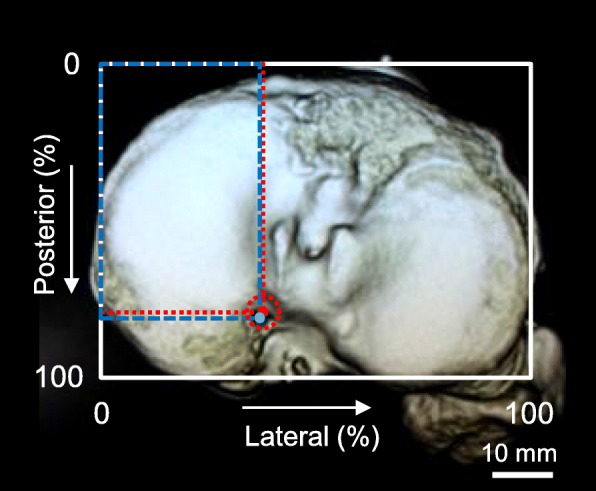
Distance between tibial tunnel center and expected anatomic center. The location on the three-dimensional computed tomography (3D CT)-based tibial surface was expressed as a posterolateral percentage using Tsukada’s method [11]. The anatomic center of the medial meniscus (MM) posterior root attachment was defined by the center of a circle (red dotted circle) that contacted three margins [anterior border of the posterior cruciate ligament (PCL) tibial attachment, lateral margin of the medial tibial plateau, and retro-eminence ridge]. Red dot, expected anatomic center of the MM posterior root attachment (in this example: 77.8% posterior and 38.5% lateral). Blue dot, tibial tunnel center (79.3% posterior and 37.1% lateral). The distance between the tibial tunnel center and anatomic center was 1.58 mm in this patient who underwent MM posterior root repair using the Unicorn Meniscal Root (UMR) guide
